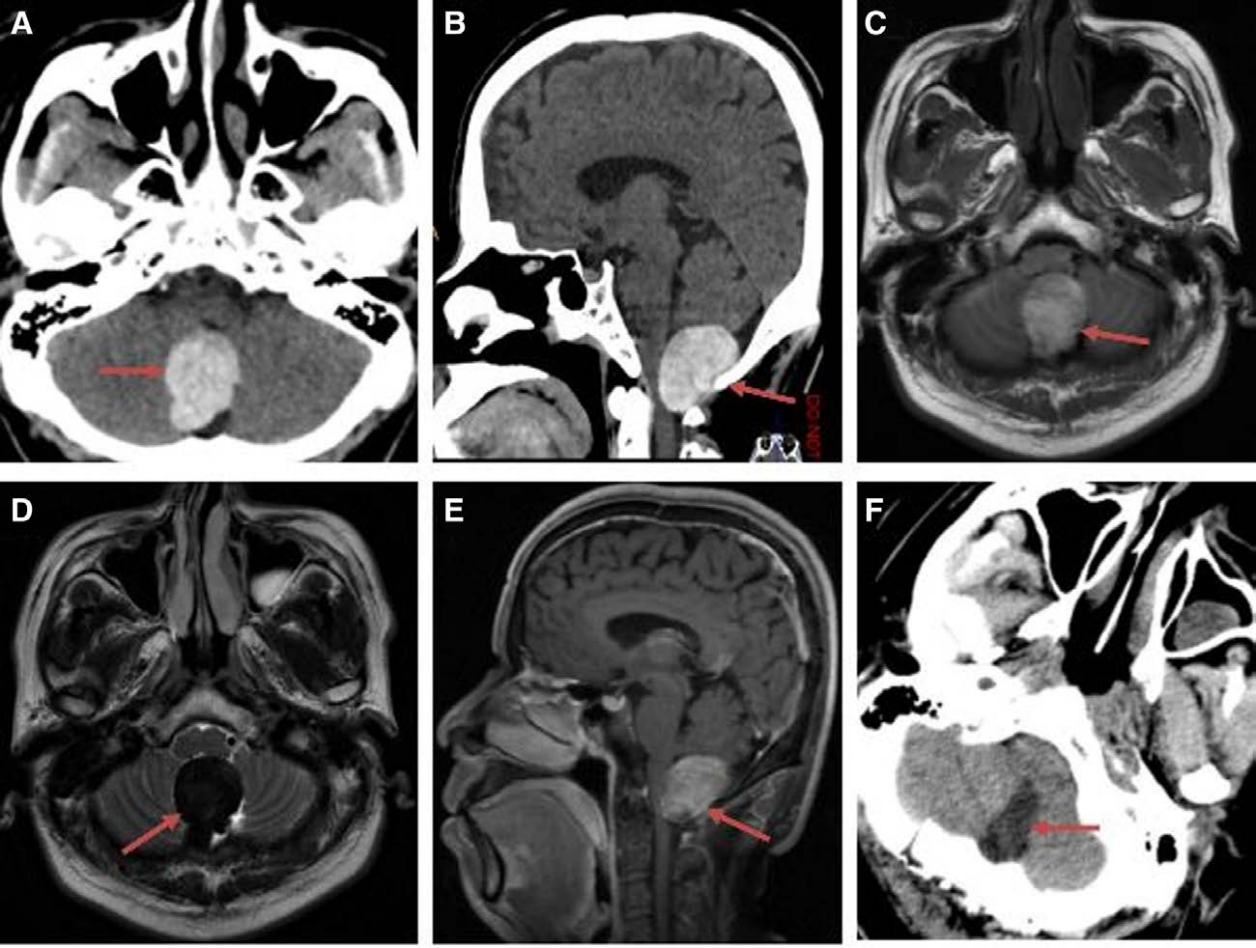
Preoperative and postoperative imaging of a 43-year-old male patient with foramen magnum melanoma. (A) Preoperative axial CT showed a high-density round lesion (red arrow). (B) Preoperative sagittal CT showed a high-density round lesion (red arrow). (C) Preoperative axial T1WI showed a high-signal round lesion (red arrow). (D) Preoperative axial T2WI showed a low-signal round lesion (red arrow). (E) Preoperative sagittal T1-weighted contrast-enhanced MRI showed a mild enhancement lesion (red arrow). (F) Axial CT at 1 day after surgery showed total tumor resection (red arrow). WI = weighted image.
